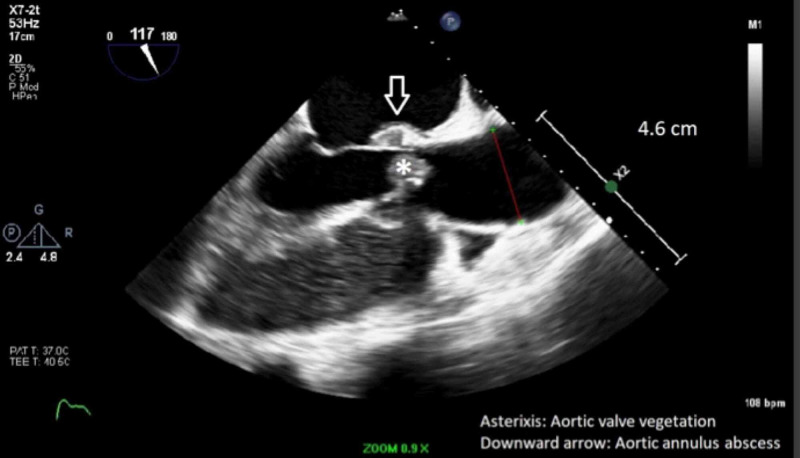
Transesophageal echocardiogram showing AV vegetation (asterisk), aortic annulus abscess (arrow) and dilated ascending aorta of 4.6 cm (red line)
